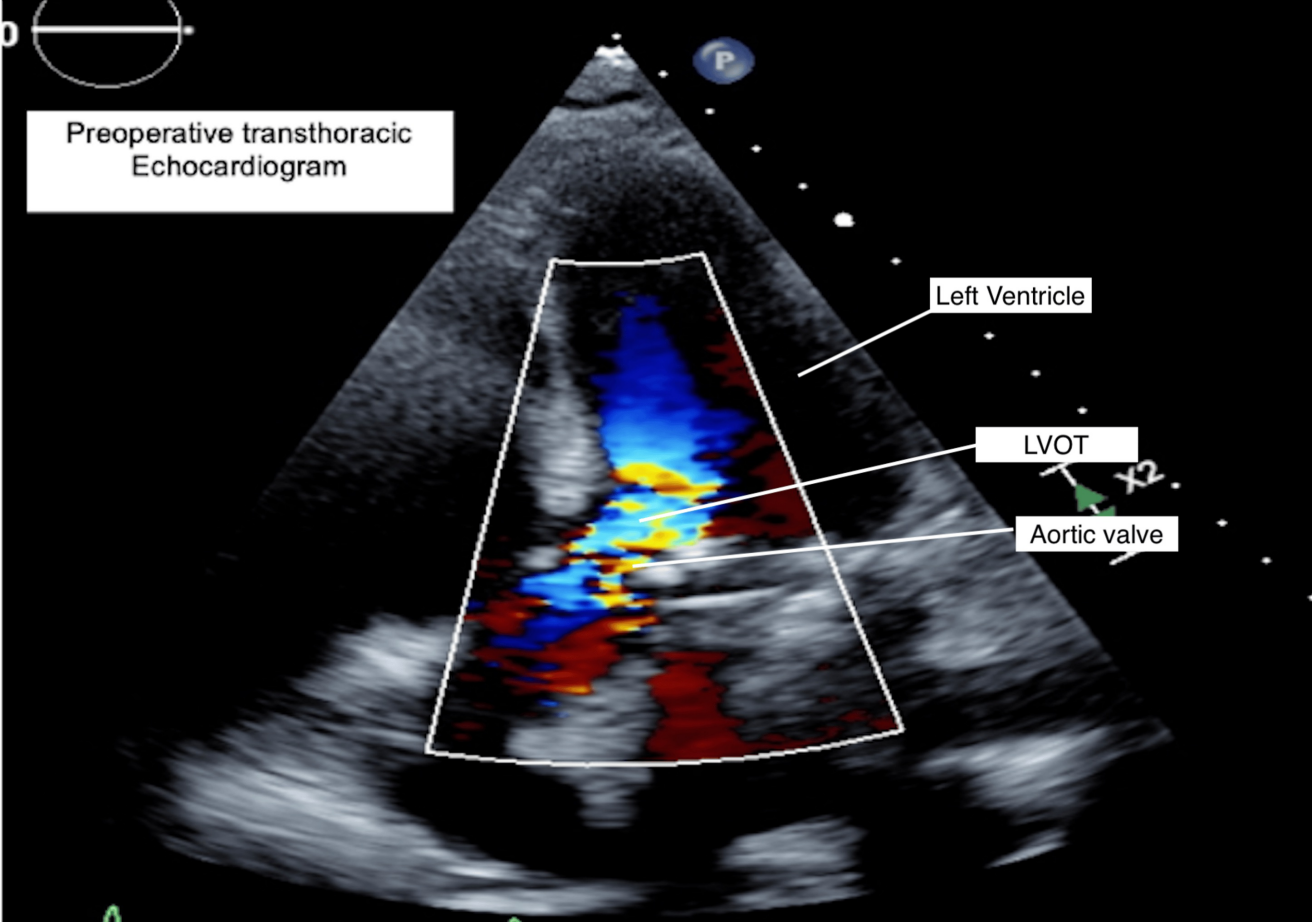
Preoperative Transthoracic echocardiogram showing LVOTO LVOTO: Left ventricular outflow tract obstruction
